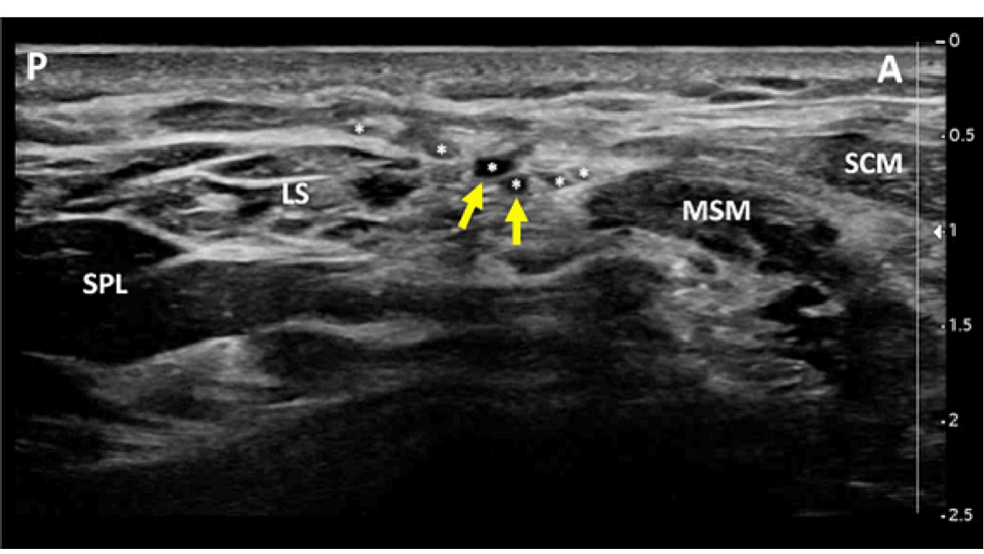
Superficial cervical plexus ultrasound imaging (transverse view) Note the superficial cervical plexus branches (asterisks) with some showing signs of enlargement and possibly neuroma formation (yellow arrows). P: posterior; A: anterior; LS: levator scapulae muscle; SPL: splenius capitis muscle; MSM: middle scalene muscle; SCM: sternocleidomastoid muscle
